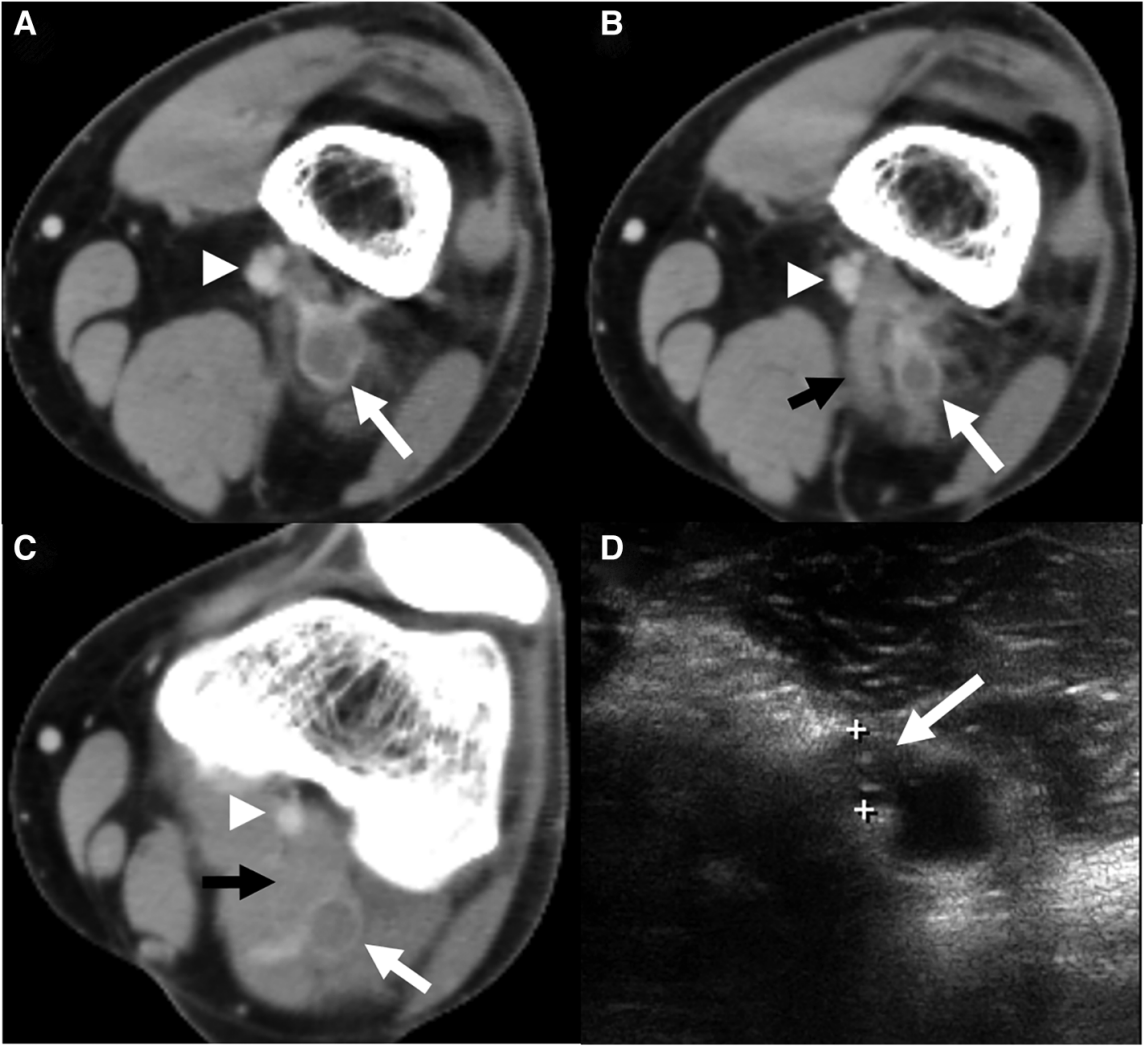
A computed tomography (CT) scan was conducted at the index visit. (A) Axial CT section revealed deep vein thrombosis (*white arrow*) of the popliteal vein (PV) with a patent popliteal artery (*arrowhead*). (B) Axial CT imaging of a point 1 cm distal showed the same findings with an abnormally inserted medial head of gastrocnemius muscle (*black arrow*) and thrombosed popliteal vein with most stenosis. (C) Axial CT of the mid portion of the popliteal fossa demonstrated thrombosed PV (*white arrow*) between both heads of the gastrocnemius muscle. (D) Duplex ultrasonography 1 year after anticoagulation demonstrated partial recanalization of the popliteal vein with residual hyperechoic fibrotic material (*white arrow*).
